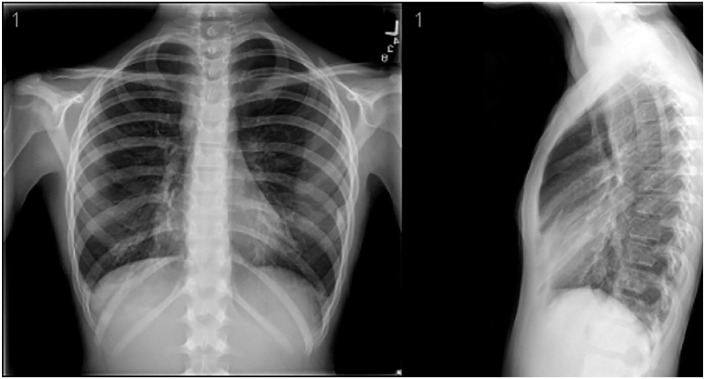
Chest x-ray with right lower lobe infiltrate.
